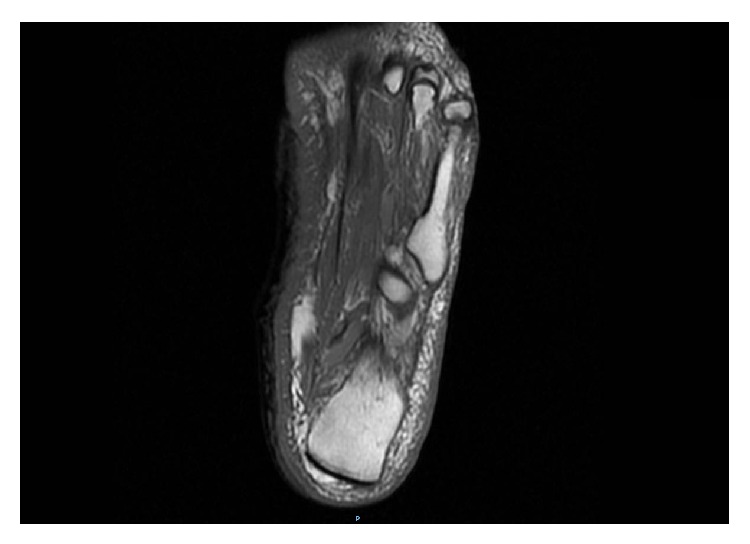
MRI of the left foot with contrast showing diffuse soft tissue T1 hypointense signal within both medial and lateral subcutaneous tissues, which is more prominent in the fat anterior to the Achilles tendon.
